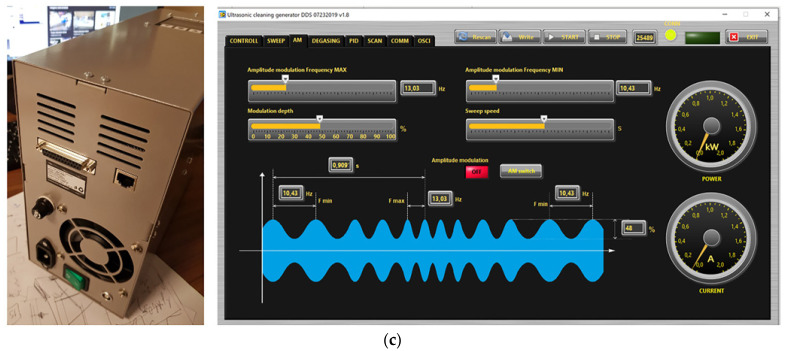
Ultrasonic caffeine extraction: (a) Laboratorial scale; (b) Pilot scale (1—Tank, 2—Chamber of extraction/homogenization, 3—Pump, 4—Mixer, 5—MMM Generator); (c) MPI Generator and software controller.
